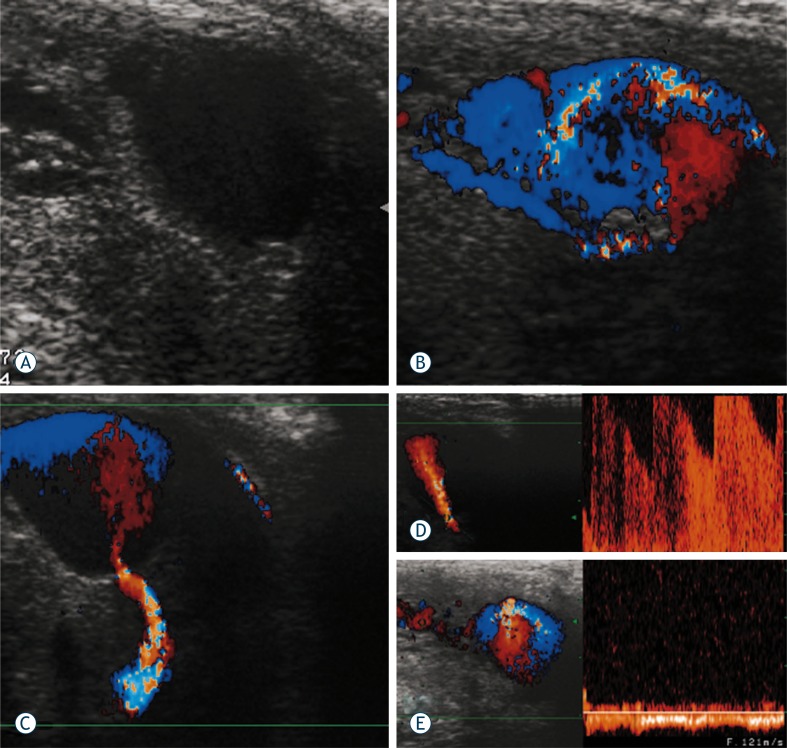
A. Gray-scale ultrasound depicts anehoic region, within corpus cavernosum. B. Colour Doppler ultrasound with multiple colour signals. C. Doppler sonogram of cavernous artery which fills the pseudoaneurysm. D. Pulsed Doppler analysis with aliasing phenomena due to turbulent high-velocity flow in the cavernous artery. E. Venous drainage in the *corpora cavenosa* on Doppler sonogram.
